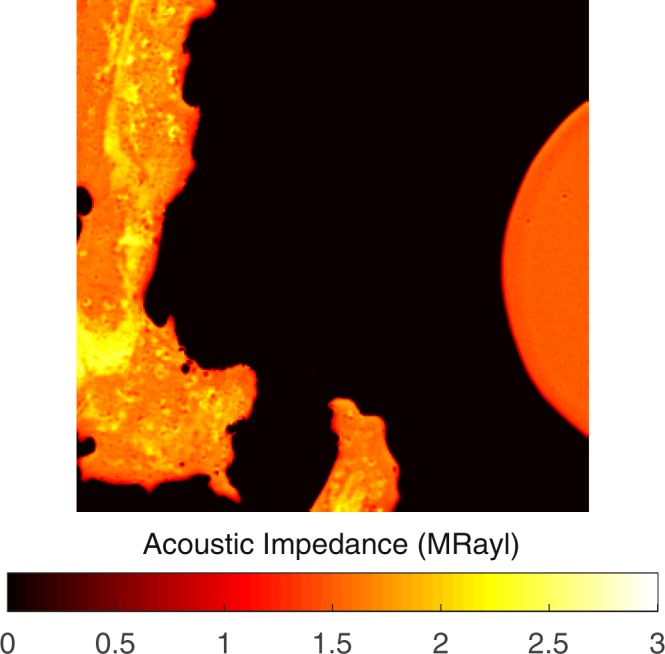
Acoustic impedance map of an atherosclerotic plaque obtained by comparing the reflected ultrasound signals from the surfaces of water and the sample. The scanning area is 4.8 mm × 4.8 mm.
